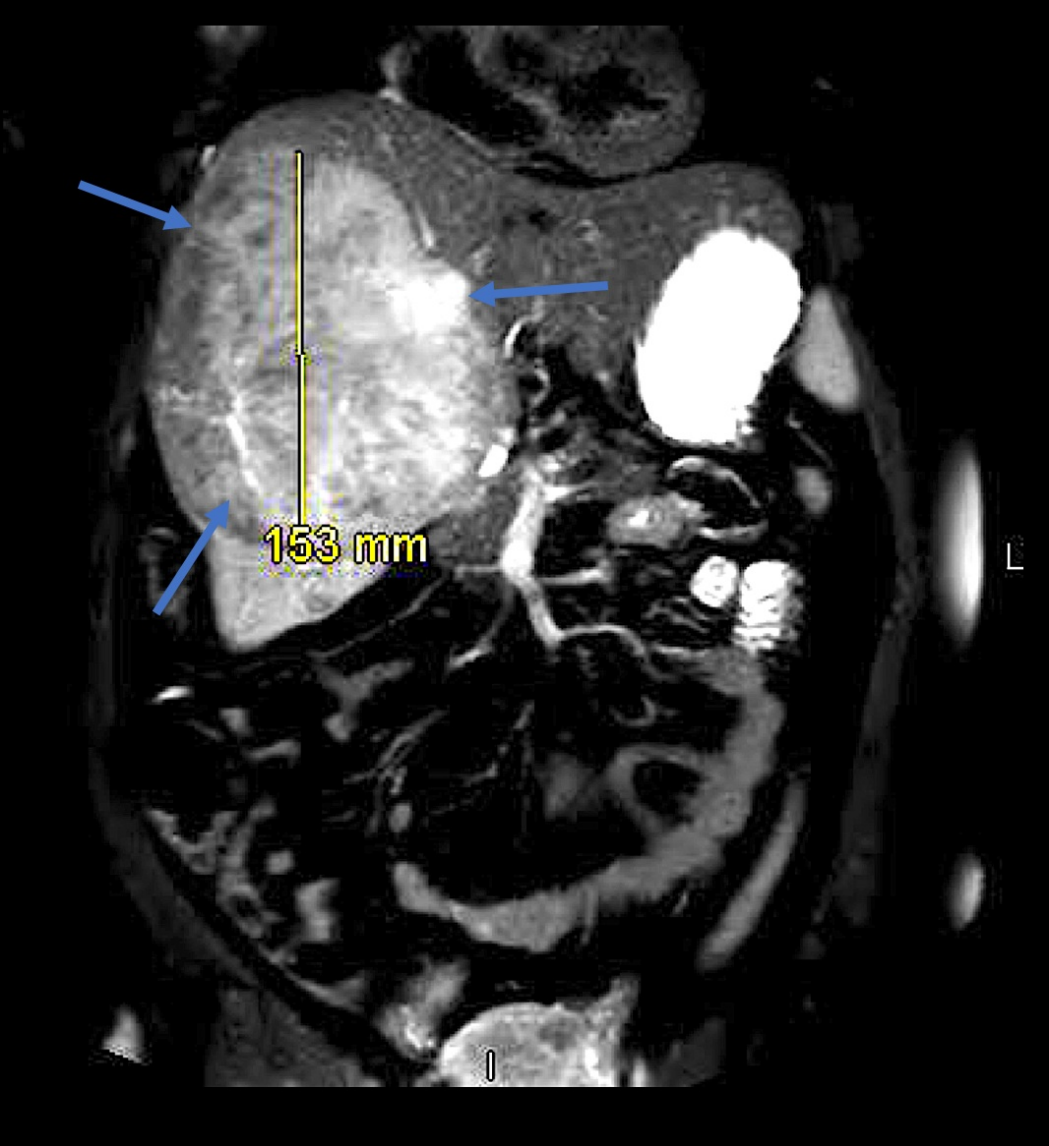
Magnetic resonance imaging of the abdomen. The magnetic resonance imaging (MRI) of the abdomen demonstrated a 15.3 cm x 12.5 cm x 14.1 cm heterogeneous mass straddling the right and left lobe of the liver. There was also peripheral enhancement consistent with hemorrhage.
